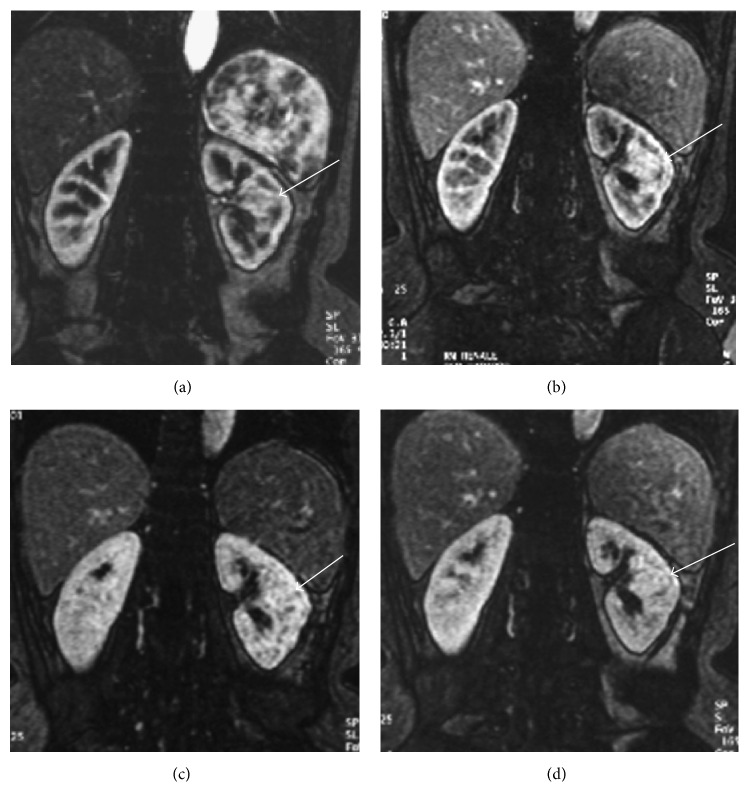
Hypertrophied column of Bertin. Coronal GE T1-weighted fat-sat gadolinium enhanced images (a–d) showing a mass-like finding in the left kidney (arrow). The prominent column of Bertin is in continuity with the renal cortex and manifests the same enhancement of the renal parenchyma in all contrastographic phases.
